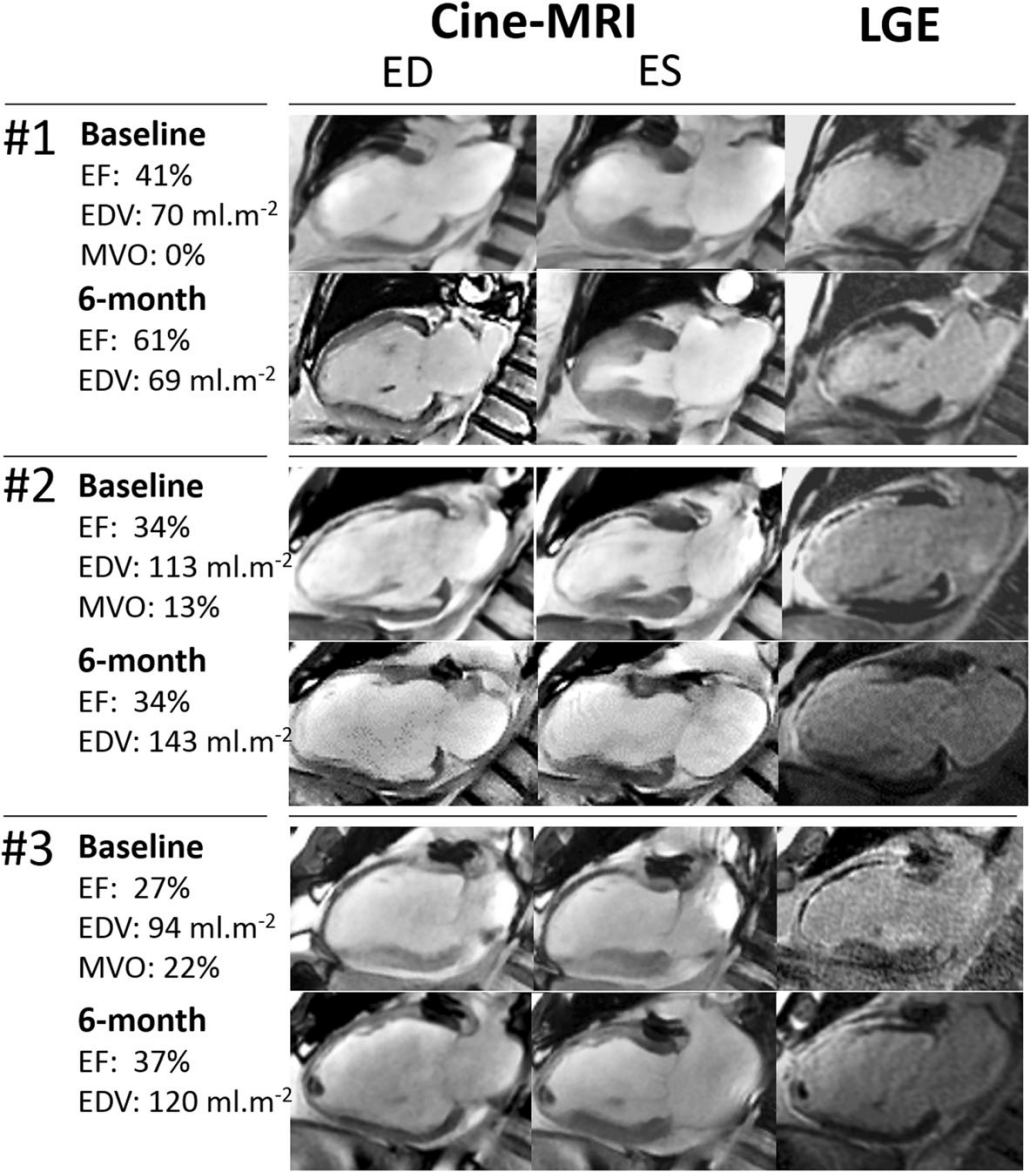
Mid-ventricular vertical long-axis slices at baseline and at 6 months obtained from late gadolinium enhancement (LGE) images (*right panel*) and cine-MRI at end-diastole (ED, *left panel*) and end-systole (ES, *middle panel*), with LV parameters (EF for ejection fraction; EDV for end diastolic volume and MVO for % of LV area with microvascular obstruction), in 3 representative cases of anterior infarction and 6-month increase(s): #1) in EF only and a 6-month decrease in TPVR (-8 mmHg.min.m^2^.L-1), #2) in EDV only and no clear change in TPVR (-1 mmHg.min.m^2^.L-1) and #3) in both EDV and EF and a 6-month decrease in TPVR (-11 mmHg.min.m^2^.L-1)
